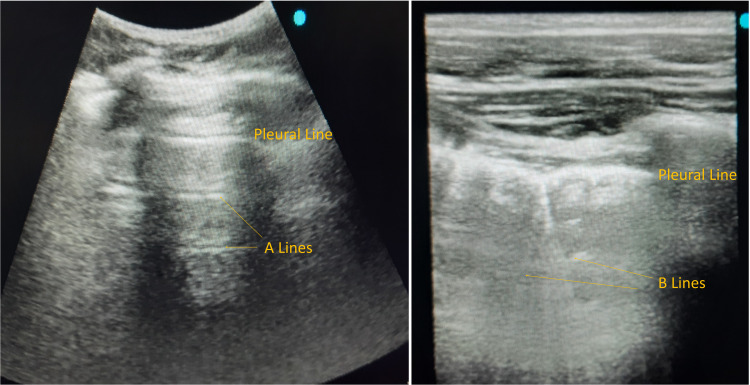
The presence of 'A' lines and 'B' lines as seen on lung ultrasound
